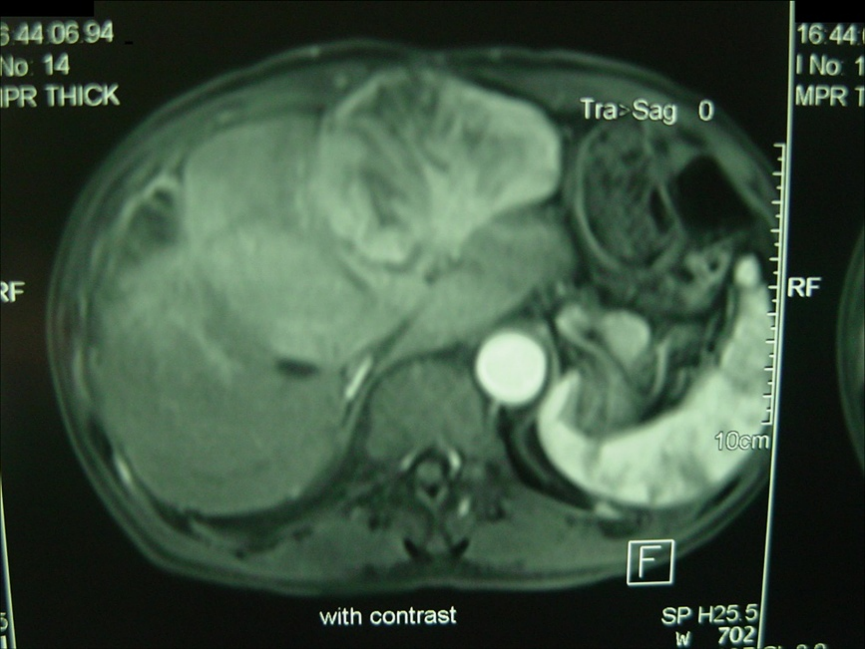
Magnetic resonance image showing recurrence of the carcinoma in segment IV after 10 months.
